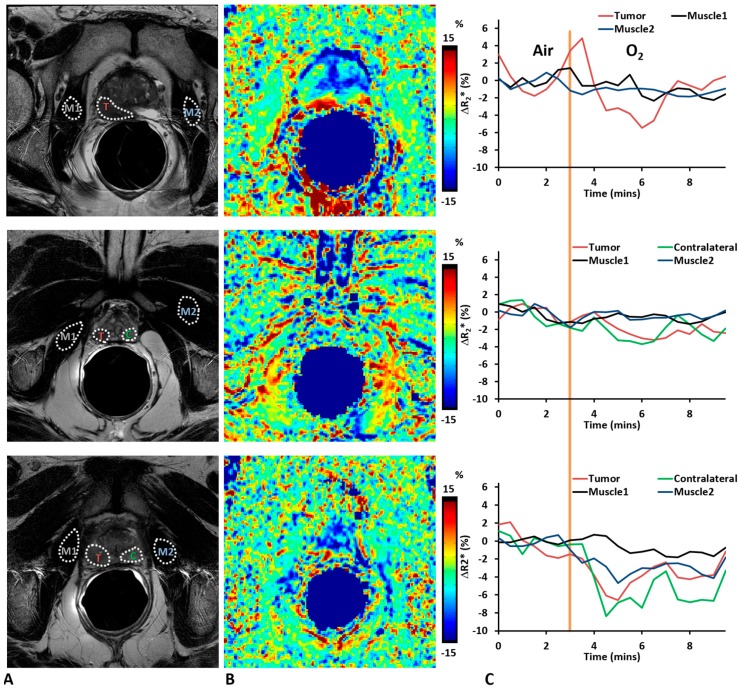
Oxygen-enhanced magnetic resonance imaging (MRI) of human prostate cancer. (A) T_2_w images showed hypointense tumor regions (T); (B) %ΔR_2_* maps (changes are normalized to baseline transverse relaxation rate (R_2_*) maps) for three representative tumors of Patients #3 (top), #5 (middle), and #6 (bottom) with Gleason scores 9, 7, and 8, respectively; (C) Traces of four regions of interest (ROIs, outlined in (A) with white dotted lines) showing dynamic changes of R_2_* in response to breathing oxygen (yellow line indicates start of oxygen breathing challenge). Tumor and normal prostate showed larger changes with oxygen breathing challenge compared to reference muscles. Labels in Figure 1A: Tumor (T), Contralateral normal prostate (C), Reference Muscle 1 and 2 (M1 and M2). Obturator internus or obturator externus muscles are selected as reference muscle. Note that in patient #3, no reliable normal prostate in the peripheral zone could be obtained due to susceptibility artefact in R_2_* maps, and the ROI of Contralateral normal prostate was omitted in this case.
